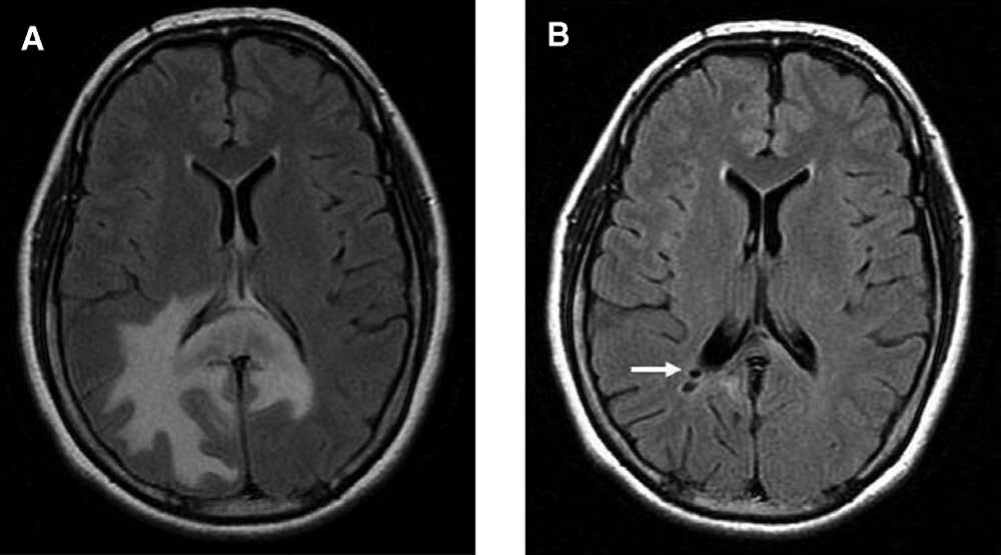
Vanishing tumor in MRI series. (A) Brain MRI showed a contrast-enhanced tumor with perifocal edema involving the corpus callosum and periventricular region. (B) Four months later, the brain tumor totally disappeared. The biopsy tract is visible (white arrow). MRI = magnetic resonance imaging.
